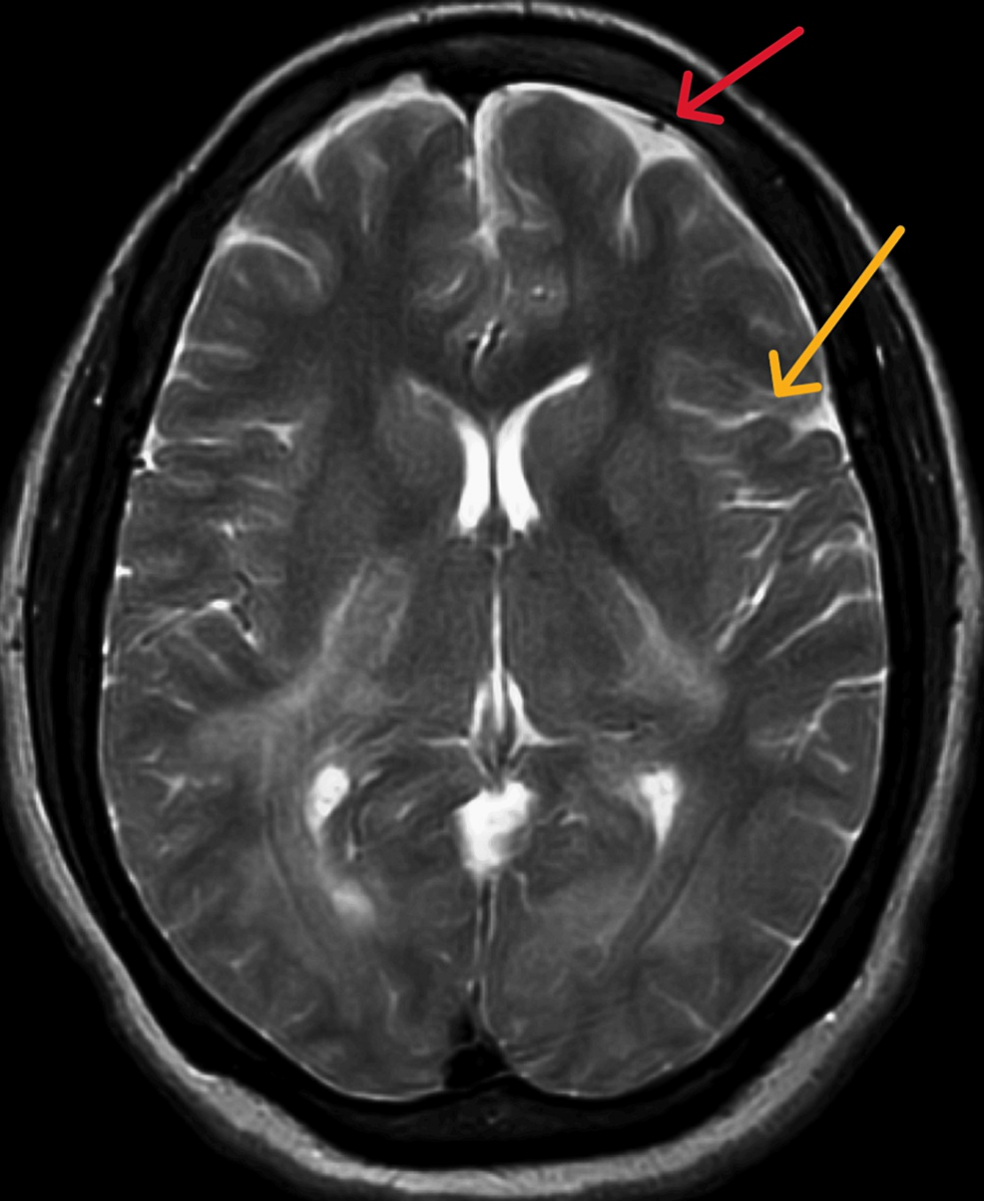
Magnetic resonance imaging (MRI) brain The red arrow denotes dural diffuse, and the yellow arrow denotes leptomeningeal markings.
